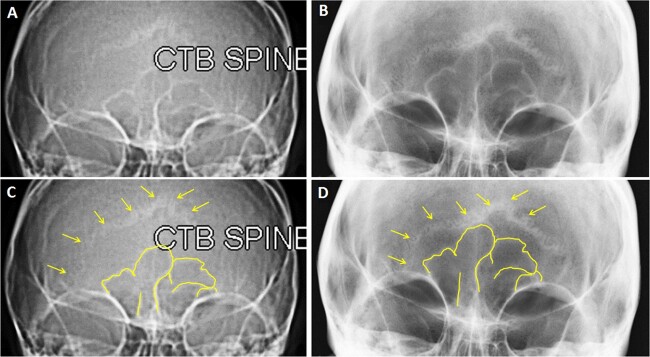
Antemortem radiographs (A, C) and postmortem radiographs (B, D) of the frontal sinus and lambdoidal suture. The frontal sinus is outlined in yellow and the lambdoidal suture is indicated by yellow arrows (C, D).
